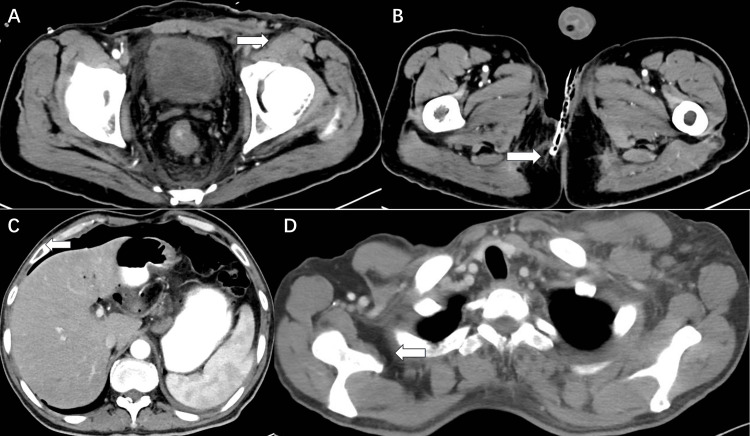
Post-treatment CT images (A-D).
